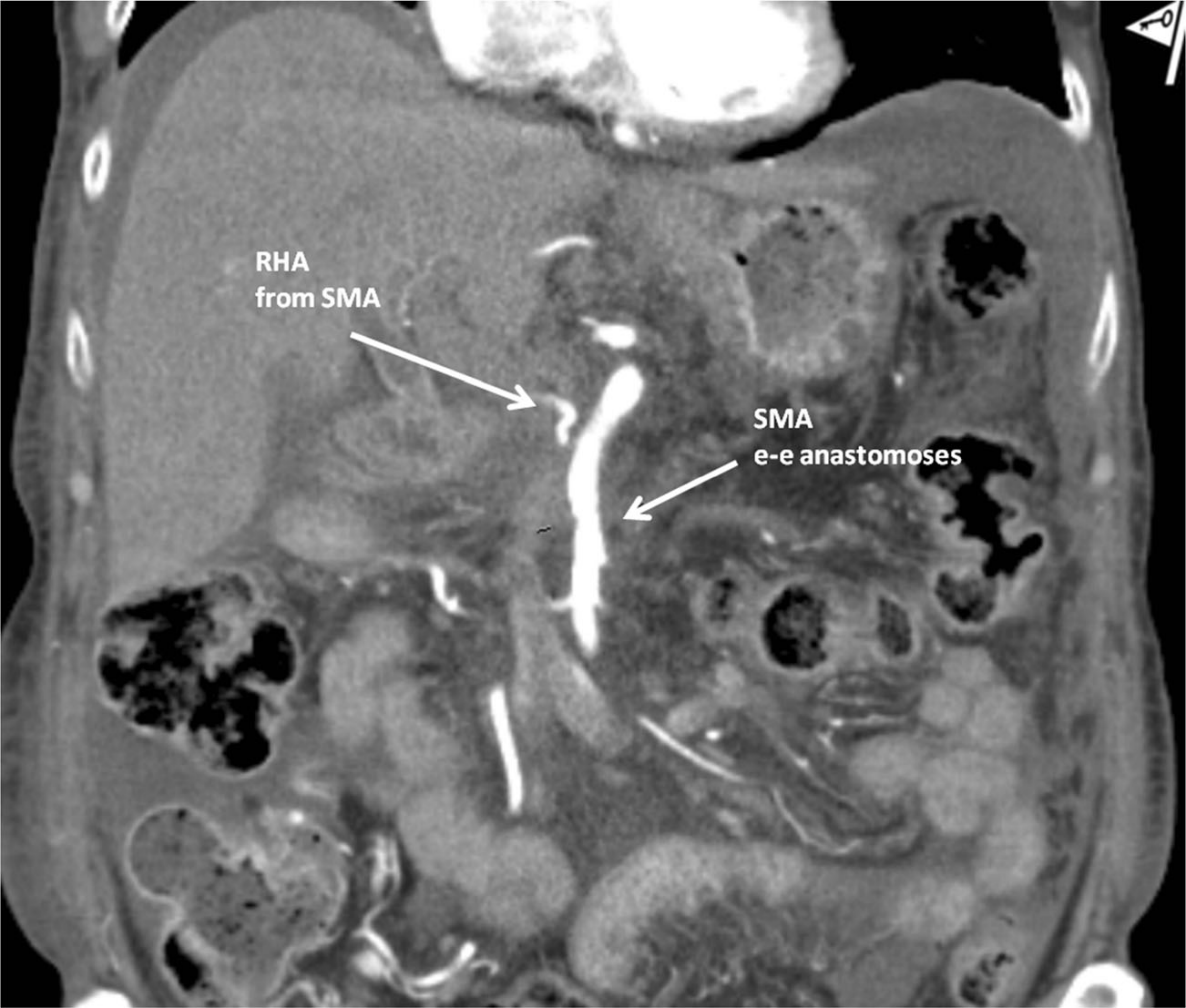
A post-operative CT scan control shows a normal canalization of the SMA anastomosisand flow in the right hepatic artery. *SMA* = superior mesenteric artery. *RHA* = right hepatic artery
